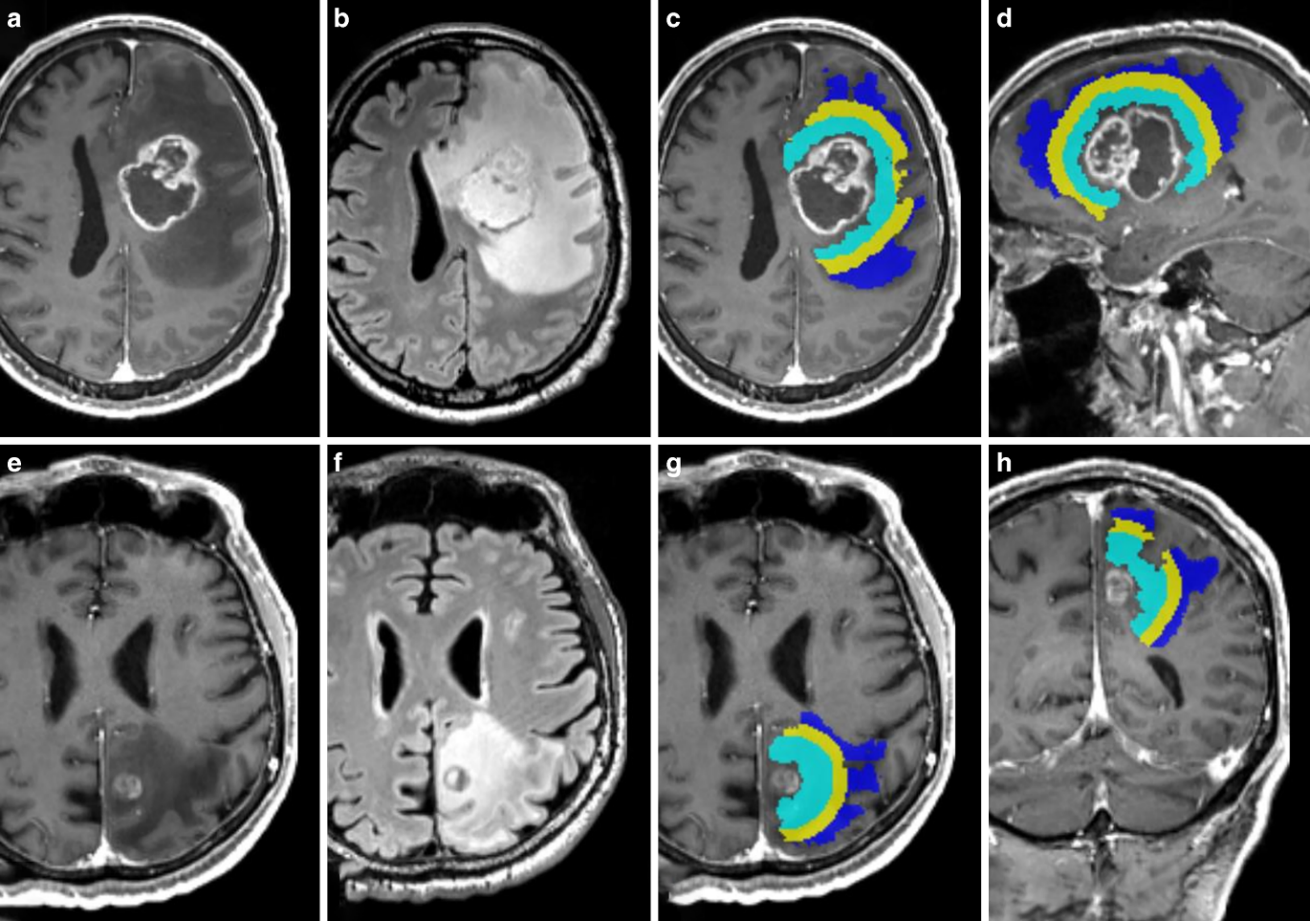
Axial T1 MPRAGE post-Gd (a,c,d,e,g,h) and FLAIR (b,f) images in a patient with a left frontal glioblastoma (upper row) and a left parietal metastasis (lower row) with corresponding color-coded subsegmentations of perilesional FLAIR-hyperintensity (c,d,g,h), parcellated in 33% zones of the total volume (innermost = IZ, turquoise; middle = MZ, yellow; and outermost zones = OZ in blue), sparing contrast-enhancing tumor components and cortex
